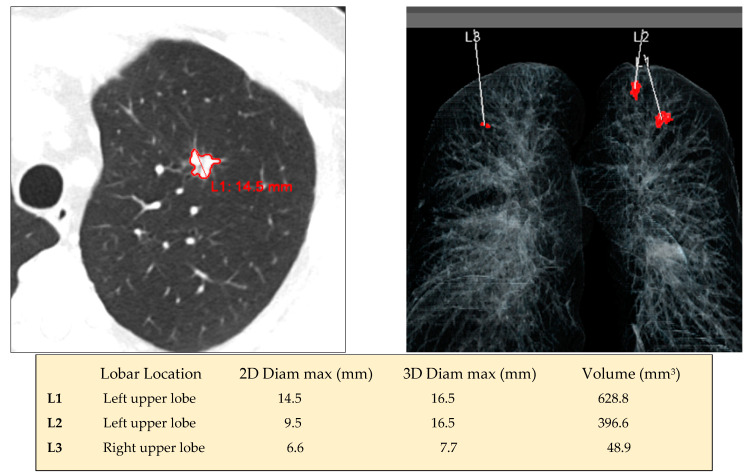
Automatic detection of 3 solid nodules in a 56 current smoker using the AI-RAD Companion (Siemens Healthinners) solution. (Left top): axial CT image that shows the results of the automatic segmentation and contouring of the largest left upper lobe nodule. (Right top): coronal view that summarizes all lung nodules detected by the CAD system with their location (red). (Bottom): table that reports the lobe location, 2D and 3D diameters, and volume of the 3 detected nodules.
